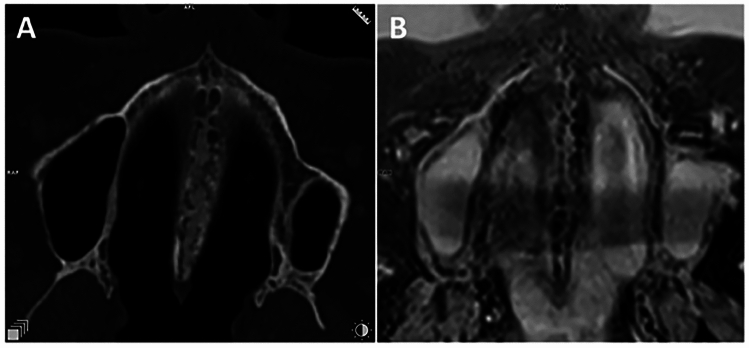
Displays the mid-palatal suture with maturation stage C. A Displays the suture in axial CT, B as an inverted image of the MRI
